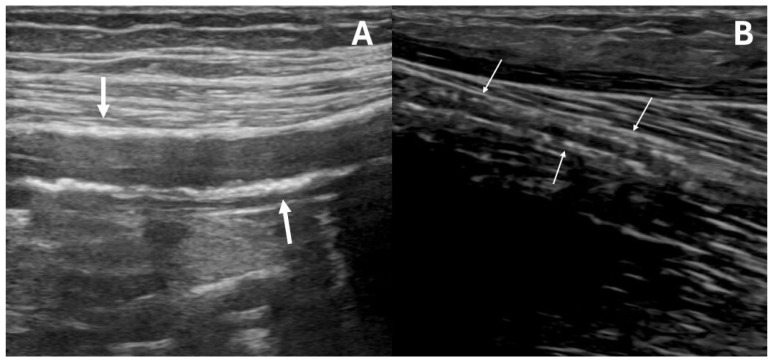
Ultrasound appearance of below-the-knee medial arterial calcification (MAC). MAC can appear as multiple hyperechogenic spots within the arterial wall (early stage, (A)) or as a continuous, hyperechogenic “railway” (late stage, (B)), as shown by the white arrows. The ultrasound image was magnified threefold (×3) and focused on its upper portion.
